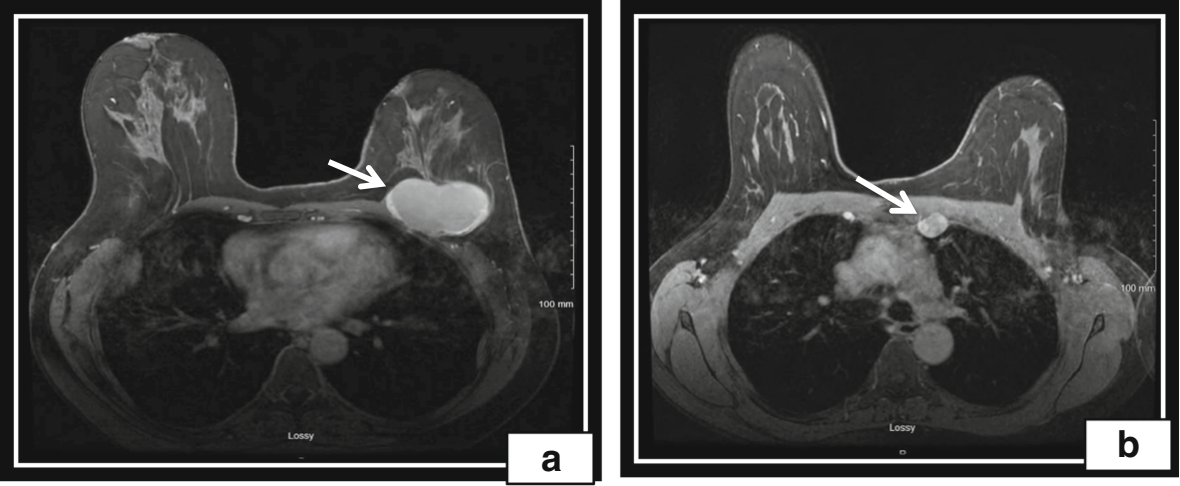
Magnetic resonance imaging of the breast with/without contrast enhancement. a Large cystic mass (white arrow) extending through the pectoralis muscle and containing a 1.8-cm area of neoplastic enhancement internally. This lesion was hypermetabolic on the positron emission tomographic scan. b An abnormal left internal mammary lymph node (white arrow) at the ​level of the sternomanubrial articulation. This was confirmed to be metastatic on the basis of biopsy
